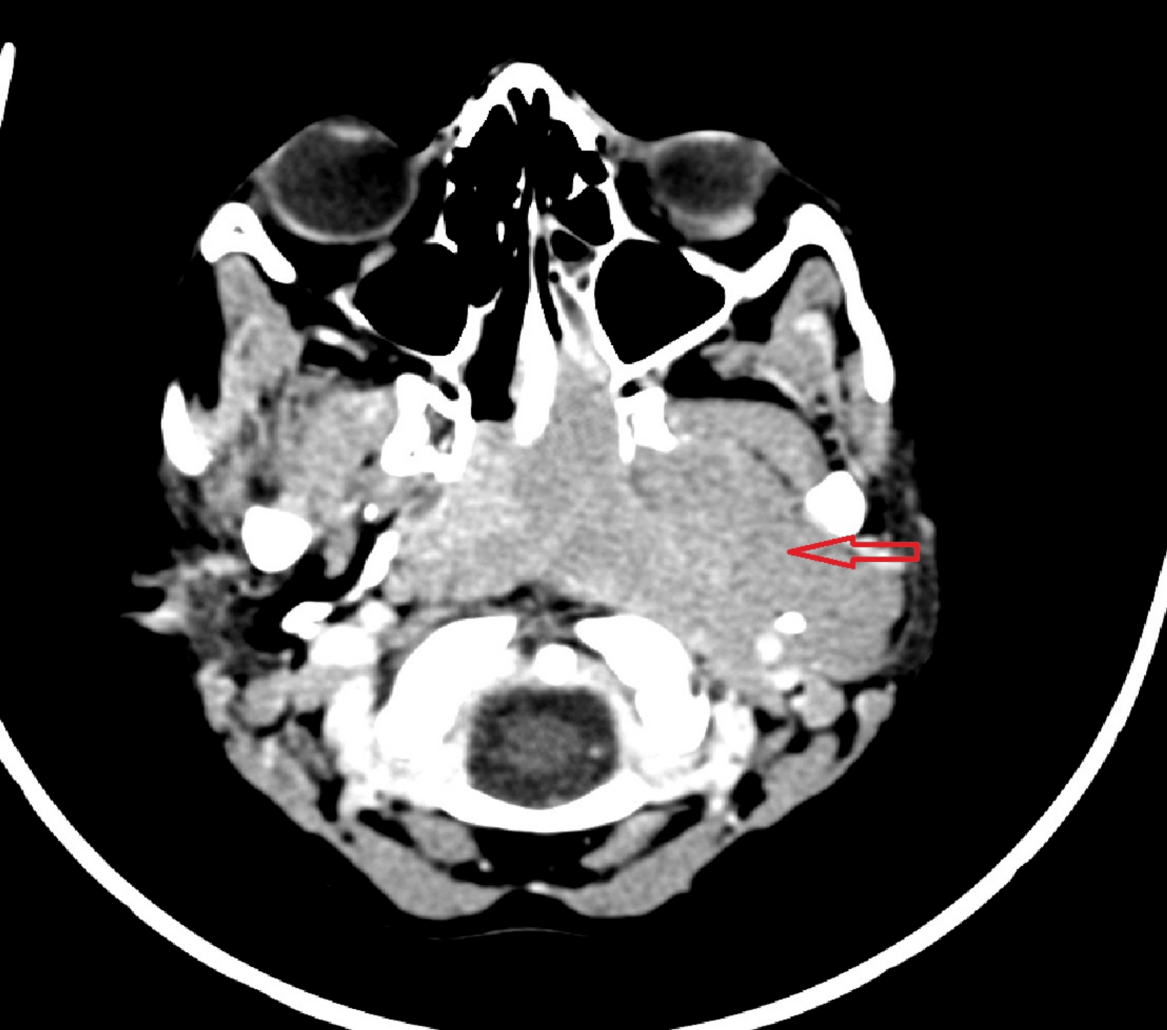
Axial CT image at the level of the nasopharynx showing a large tumor mass (red arrow) extending anteriorly toward the choanae and laterally invading the left masticator space.
